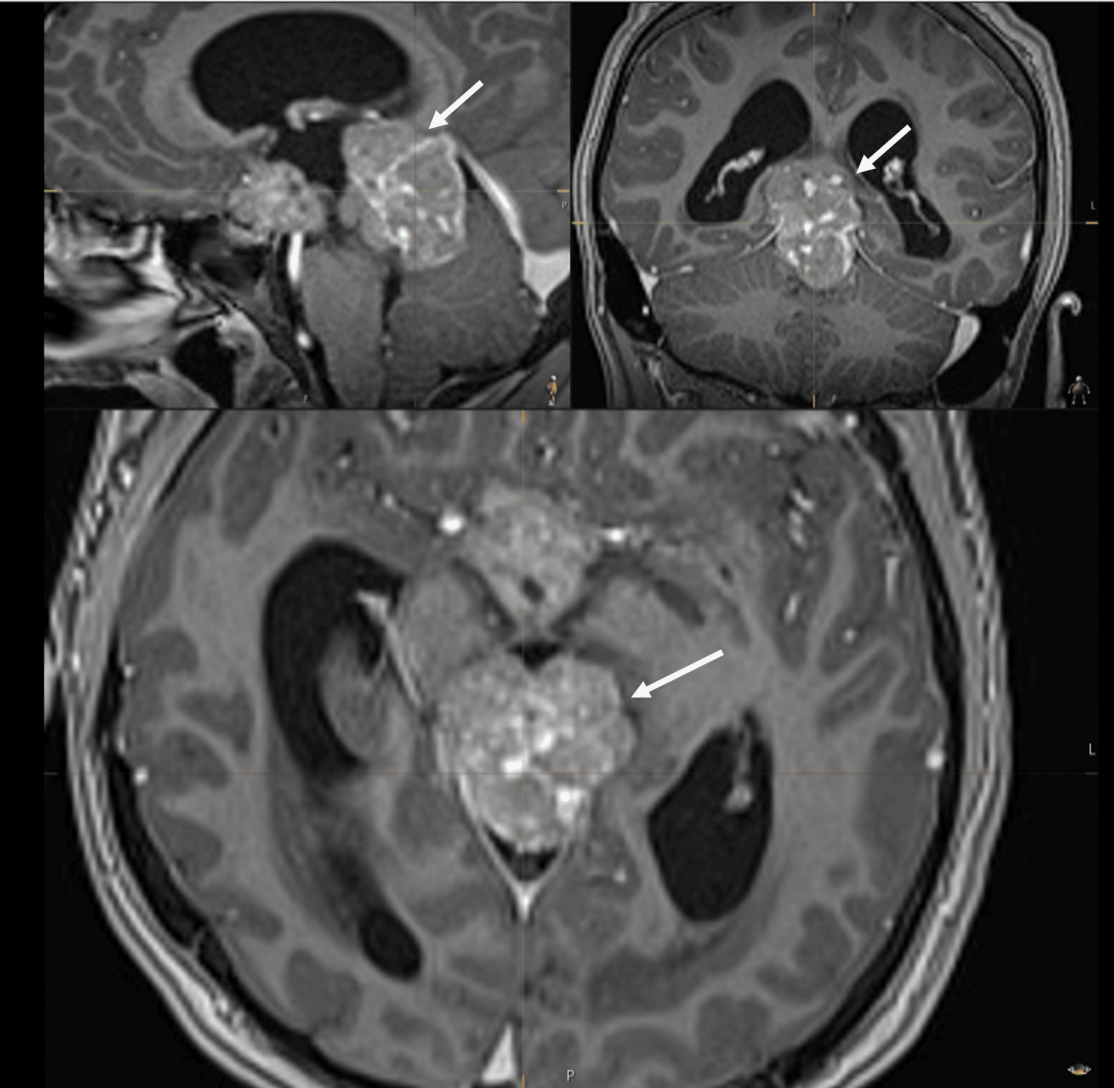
MRI of the head with contrast. White arrows: mass lesion in the pineal region.
